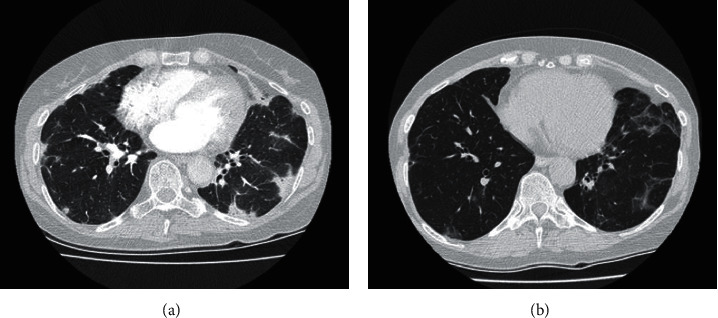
Imaging studies of the patient. (a) CT-thorax 2018 (pretreatment): bilateral patchy consolidations with distortion and dilatation of the bronchioles and parenchymal distortion with peripheral subpleural and peribronchovascular distribution, which are compatible with an organizing pneumonia. (b) CT-thorax 2019 (posttreatment): bilateral atelectasis with traction bronchiectasis, which are compatible with residual abnormalities after an organizing pneumonia.
